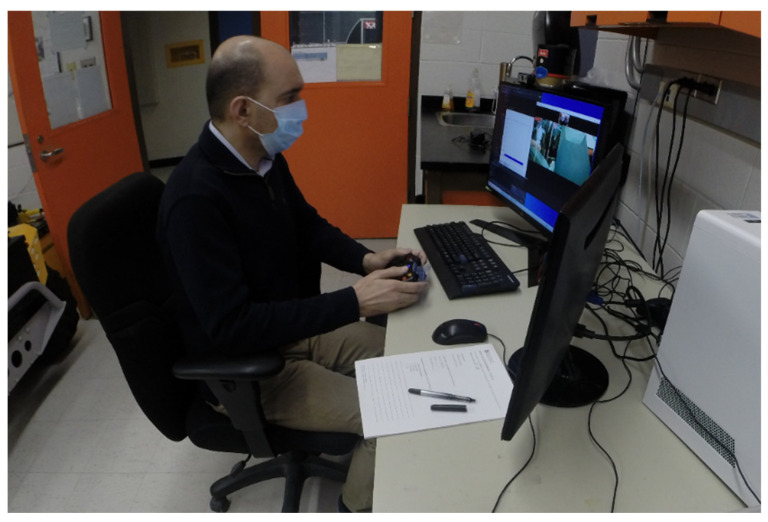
Setup of the master station for the musculoskeletal telesonography system.
